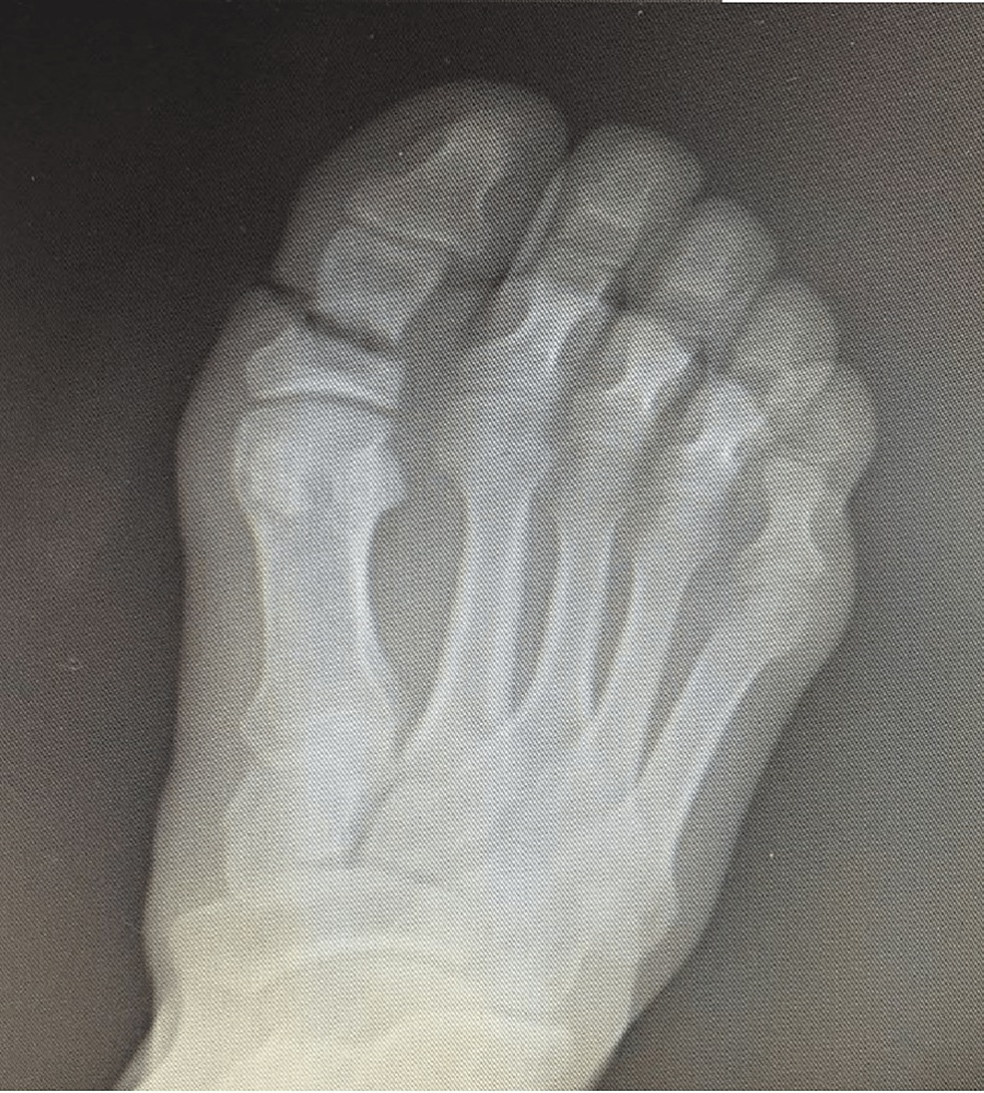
Subtotal toe amputation, AP radiograph
